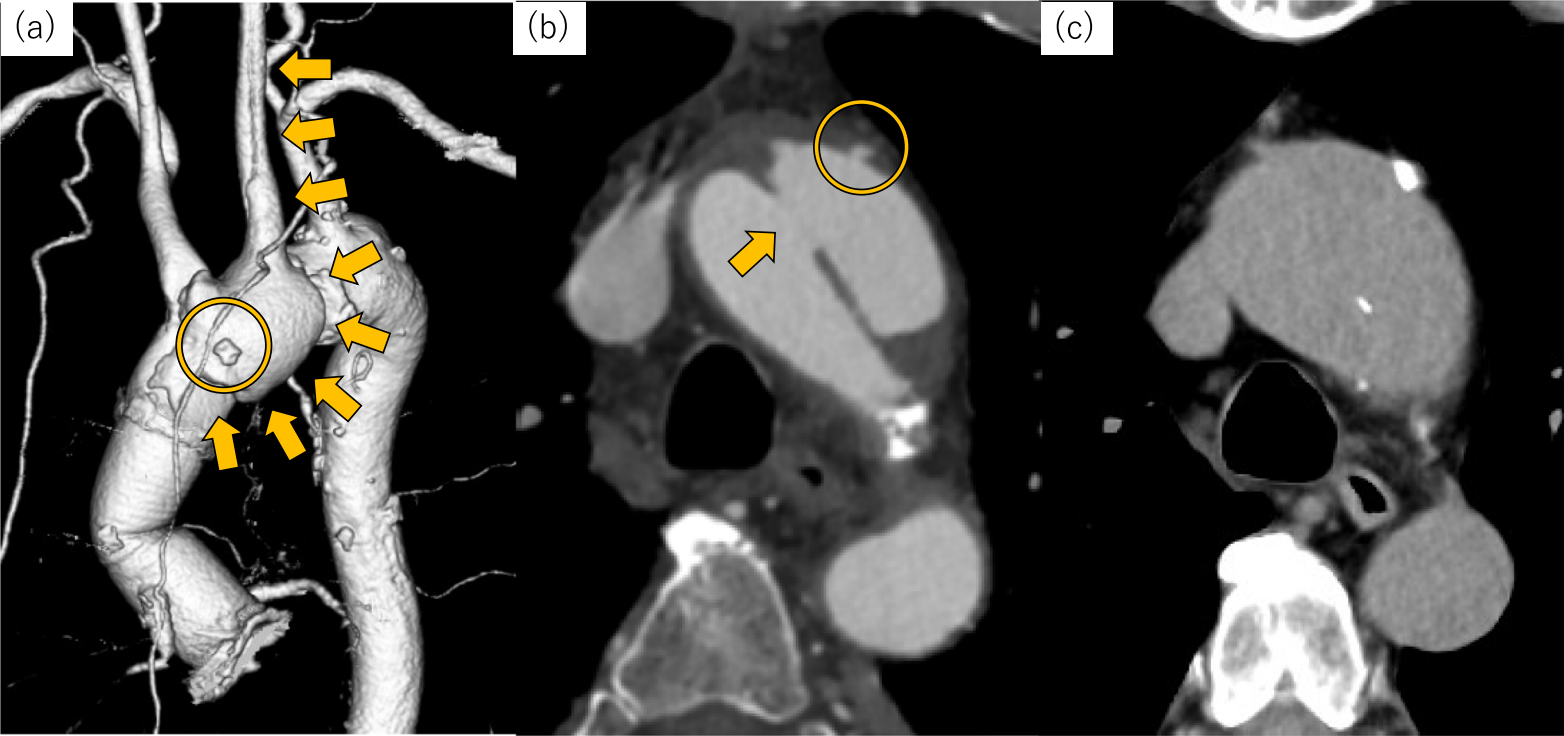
Contrast-enhanced CT at POD 14 (a, b) and plain CT at 6 months after surgery (c). a Postoperative Stanford type A aortic dissection (arrows). Dissection was extended to the left common carotid artery. Felt pledget is located at the aortic cannulation site (circle). The aortic root had been replaced (asterisk). b The entry was at the distal ascending aorta (arrow) just below the felt pledget at the cannulation site (circle). c The aortic diameter was not changed
